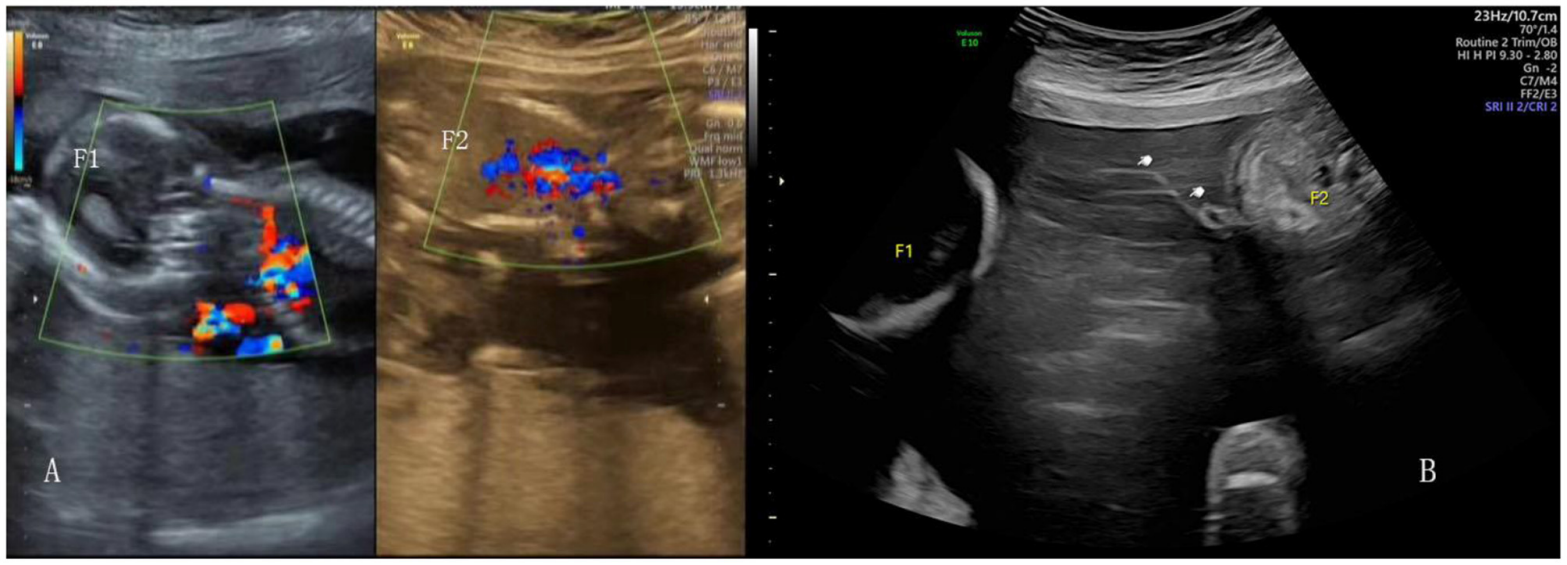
Ultrasound image of the surviving twin after RFA (A). Ultrasound image of TTTS, the donor amnion (white hand) floating in the amniotic cavity (B).
